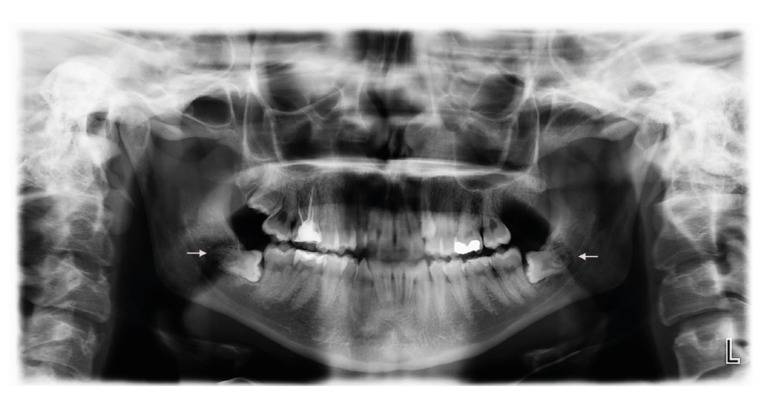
Orthopantomogram of a representative patient.
